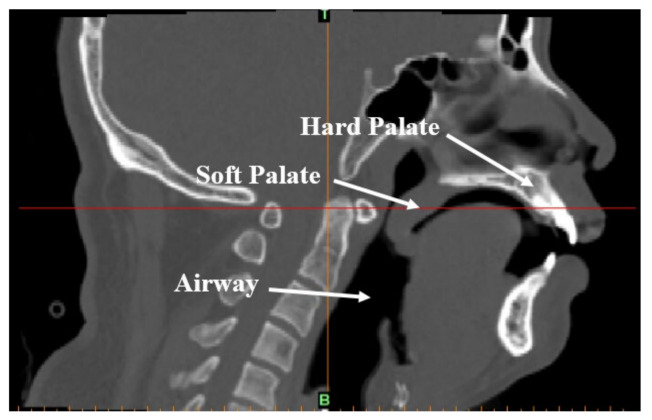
CT-scanned image of the upper airway of an OSAHS patient in a supine position and sleeping naturally.
